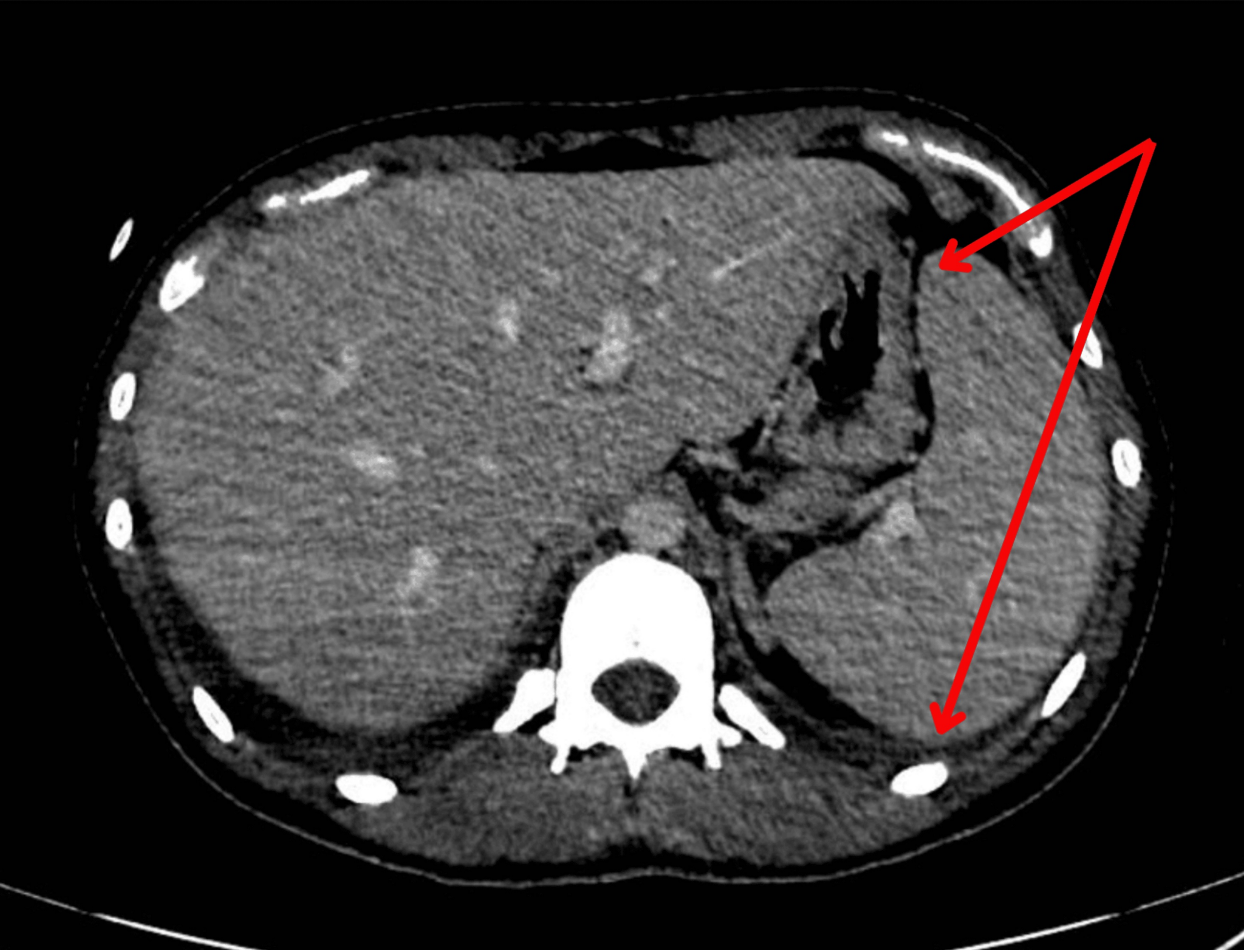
CT of the abdomen showing mild splenomegaly.
